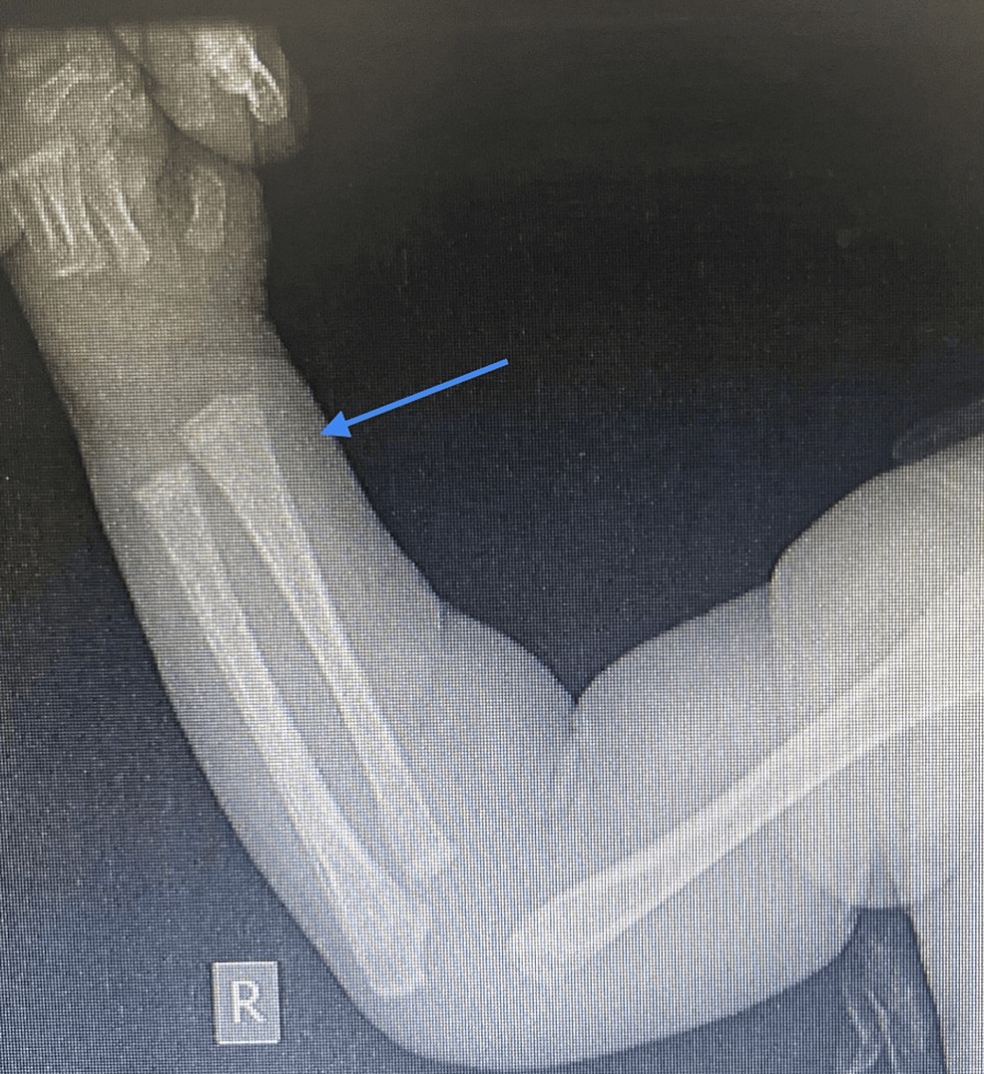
X-ray scan of periosteal reaction in the right radius.
